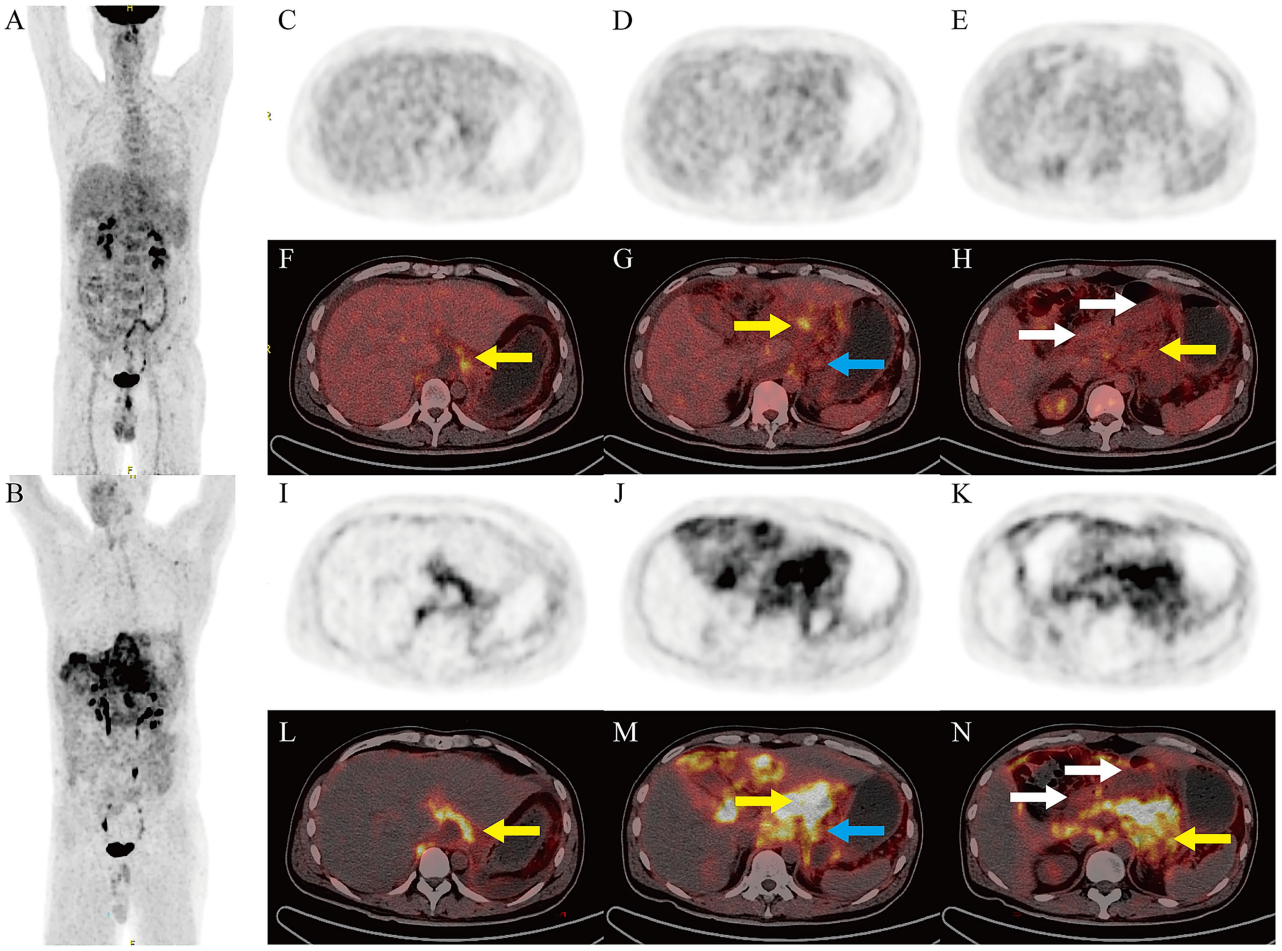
PET/CT Imaging. Maximum intensity projection of ^18^F-FDG (A) and ^18^F-FAPI (B) PET. Axial ^18^F-FDG PET (C–E) and PET/CT fusion scans (F–H), axial ^18^F-FAPI PET (I–K), and PET/CT fusion scans (L–N).
